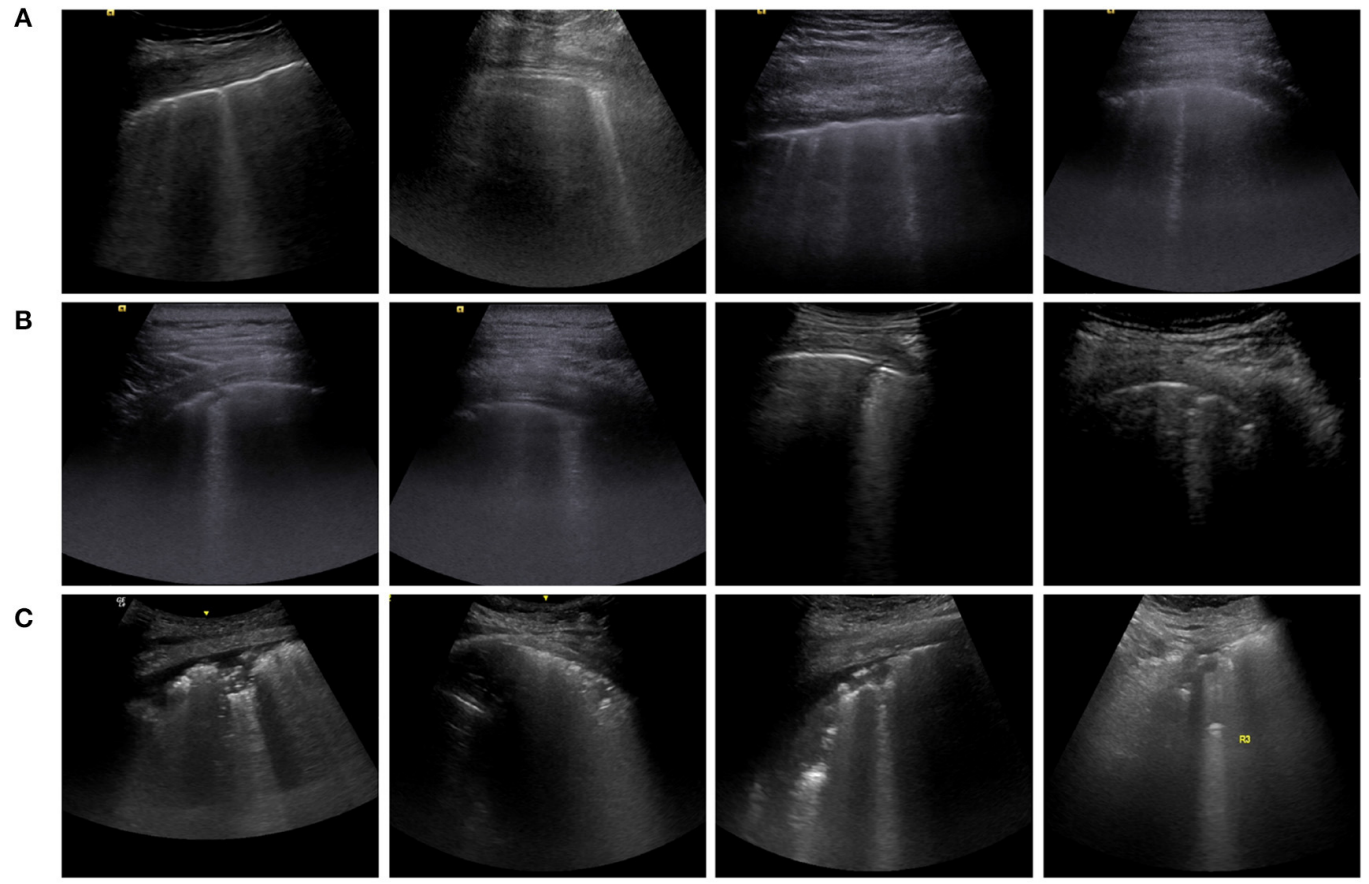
Typical examples of abnormal ultrasound cases related to coronavirus disease 2019 (COVID-19). (A) The pleural line is jagged or concave. (B) The pleural line is broken. (C) The scanning area shows a wide range of dense white areas, with or without large consolidation.
